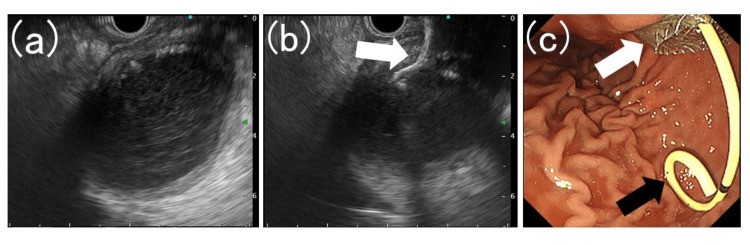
Placement of a lumen-apposing metal stent in the peripancreatic fluid collection and a double-pigtail stent in the lumen (a) Pancreatic fluid collection is observed in the stomach using an ultrasound endoscope. (b) Lumen-apposing metal stent (white arrow) is deployed by puncturing the peripancreatic fluid collection from the stomach under endoscopic ultrasound guidance. (c) A double-pigtail plastic stent (black arrow) was placed in the lumen of the lumen-apposing metal stent (white arrow).
